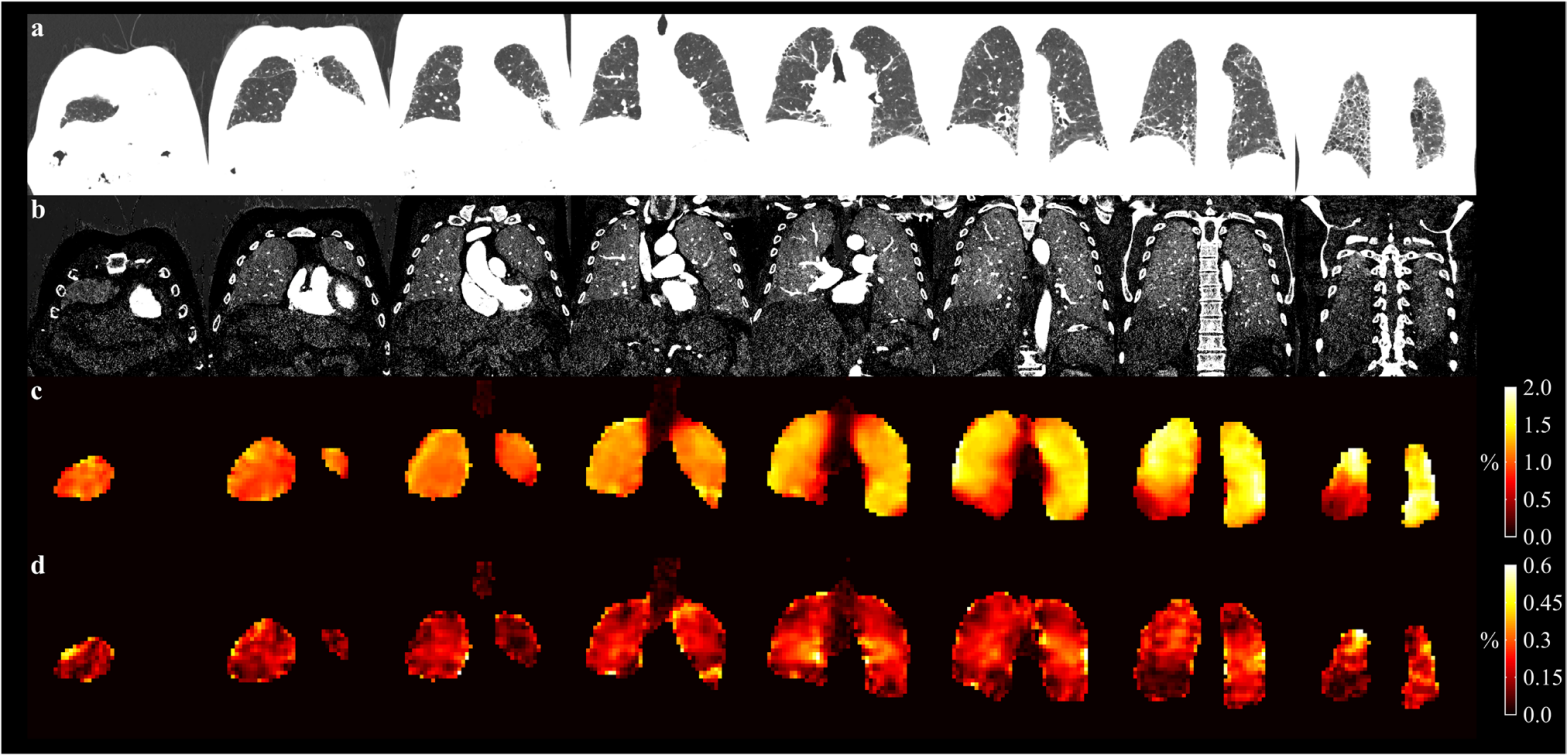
(a) Anatomical virtual native CT images and (b) iodine maps in similar slice location as ^129^Xe dissolved‐phase ratio maps for M‐Gas (c) and RBC‐Gas (d) in a 73‐year‐old male patient with idiopathic pulmonary fibrosis. Heterogeneity of gas uptake is observed in both ratio maps. RBC, red blood cell, M, membrane tissues.
